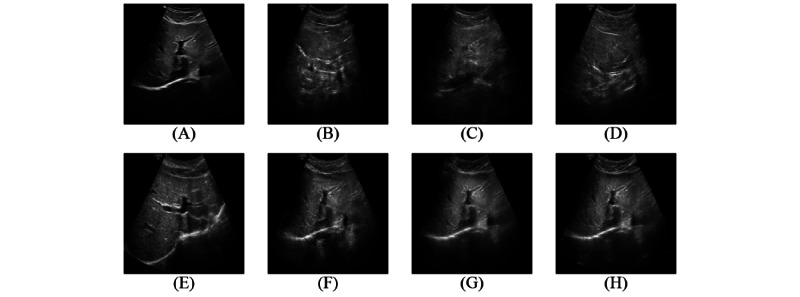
A comparative visualization of ultrasound scans from the test set, showcasing the performance of different enhancement frameworks on the same high-low quality image pair. Each subfigure corresponds to a different model’s output, allowing for a direct comparison of the anatomical preservation and image quality achieved by each approach. (A) reference low, (B) Pix2Pix [27], (C) MSPGAN [11], (D) RegGAN [23], (E) reference high, (F) CycleGAN [13], (G) stable CycleGAN [12], and (H) proposed method. CycleGAN: cycle-consistent generative adversarial network; MSPGAN: multilevel structure-preserved generative adversarial network; RegGAN: registration generative adversarial network.
